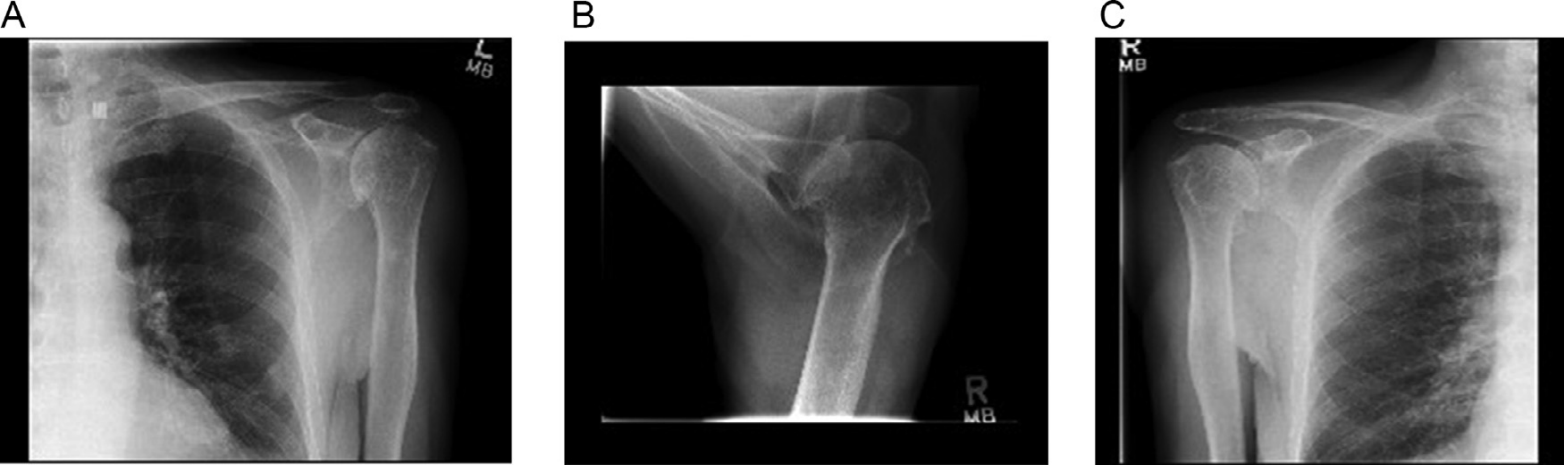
Bilateral shoulder radiographs obtained 1 month before cryoneurolysis show right sided moderately severe degenerative change and left moderate to severe arthritic change with flattening of the humeral head and prominent inferior osteophytes. (A) Frontal plane view of the left shoulder. (B) Axillary view of the right shoulder. (C) Frontal plane view of the right shoulder.
